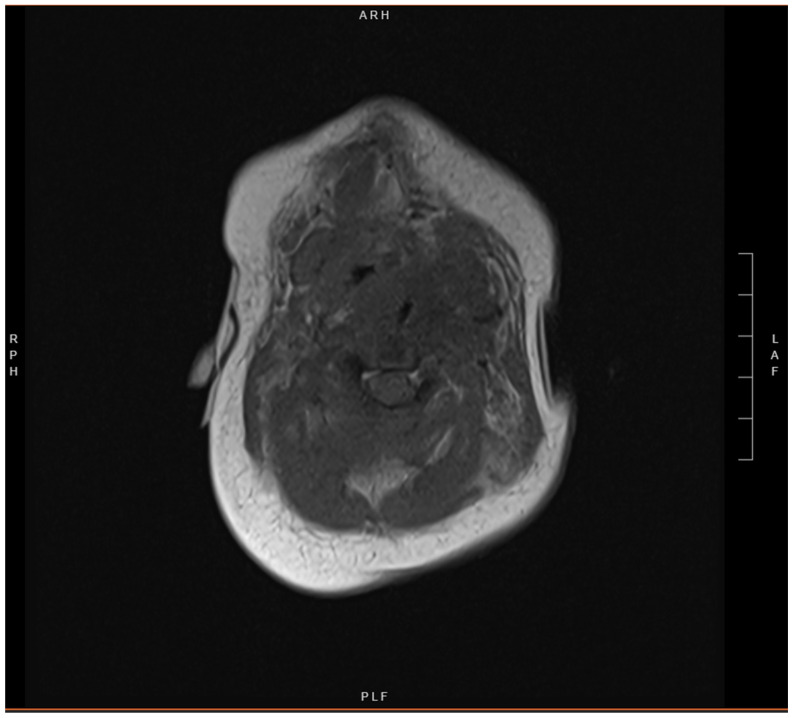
Axial short tau inversion recovery (STIR) MRI image demonstrating post-surgical changes at the level of the larynx from interval resection.
